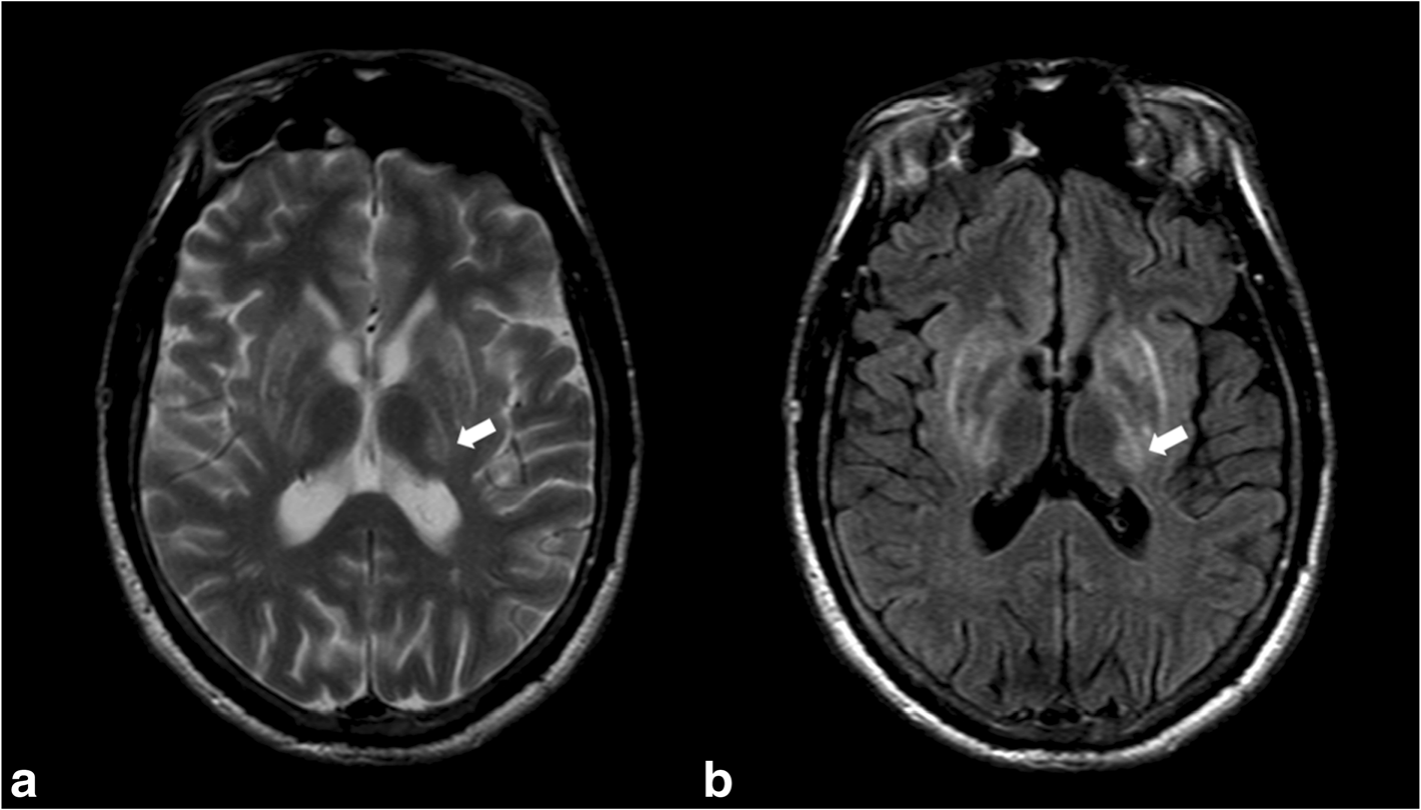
High signal intensity of the basal ganglia and left thalamus (arrows) on FSE T2WI (a) and FLAIR (b) in a patient known for Wilson’s disease
